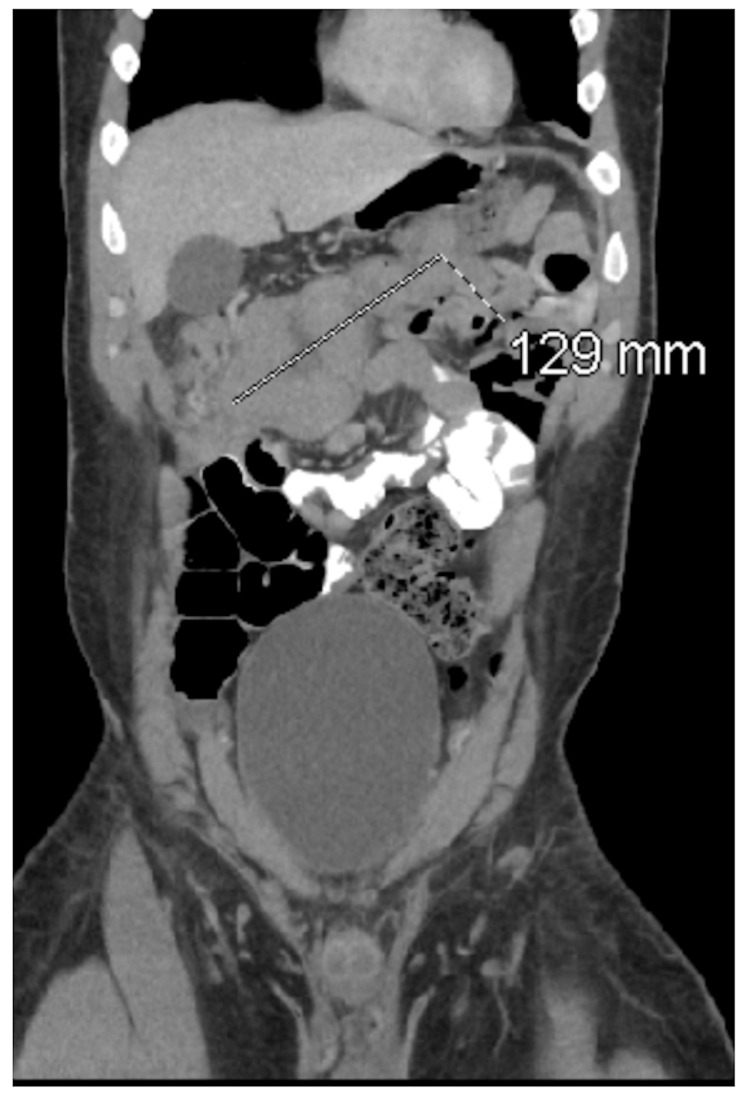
CT with contrast with coronal view showing a 12.9 cm mass at the hepatic flexure
